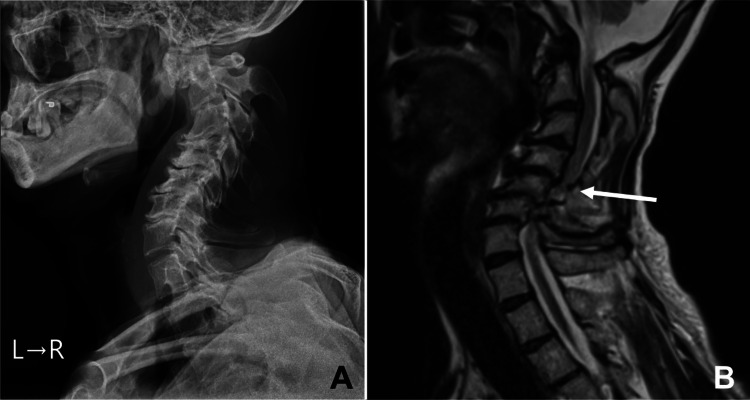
Preoperative radiological images. A lateral projection of the X-ray image shows cervical kyphosis (A). Severe cervical spinal cord compression can be observed (white arrow) (B).
